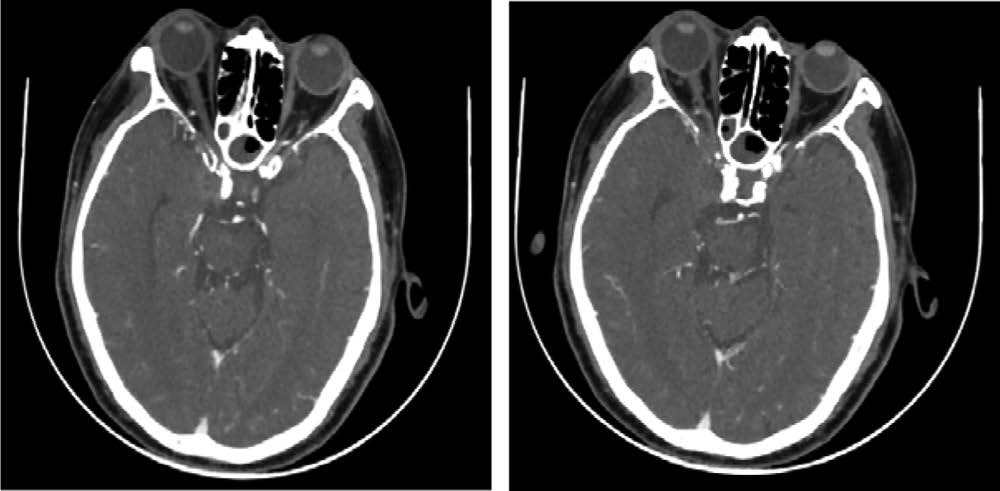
Postoperative CT angiogram showing the disappearance of pseudoaneurysm. CT = computed tomography.
